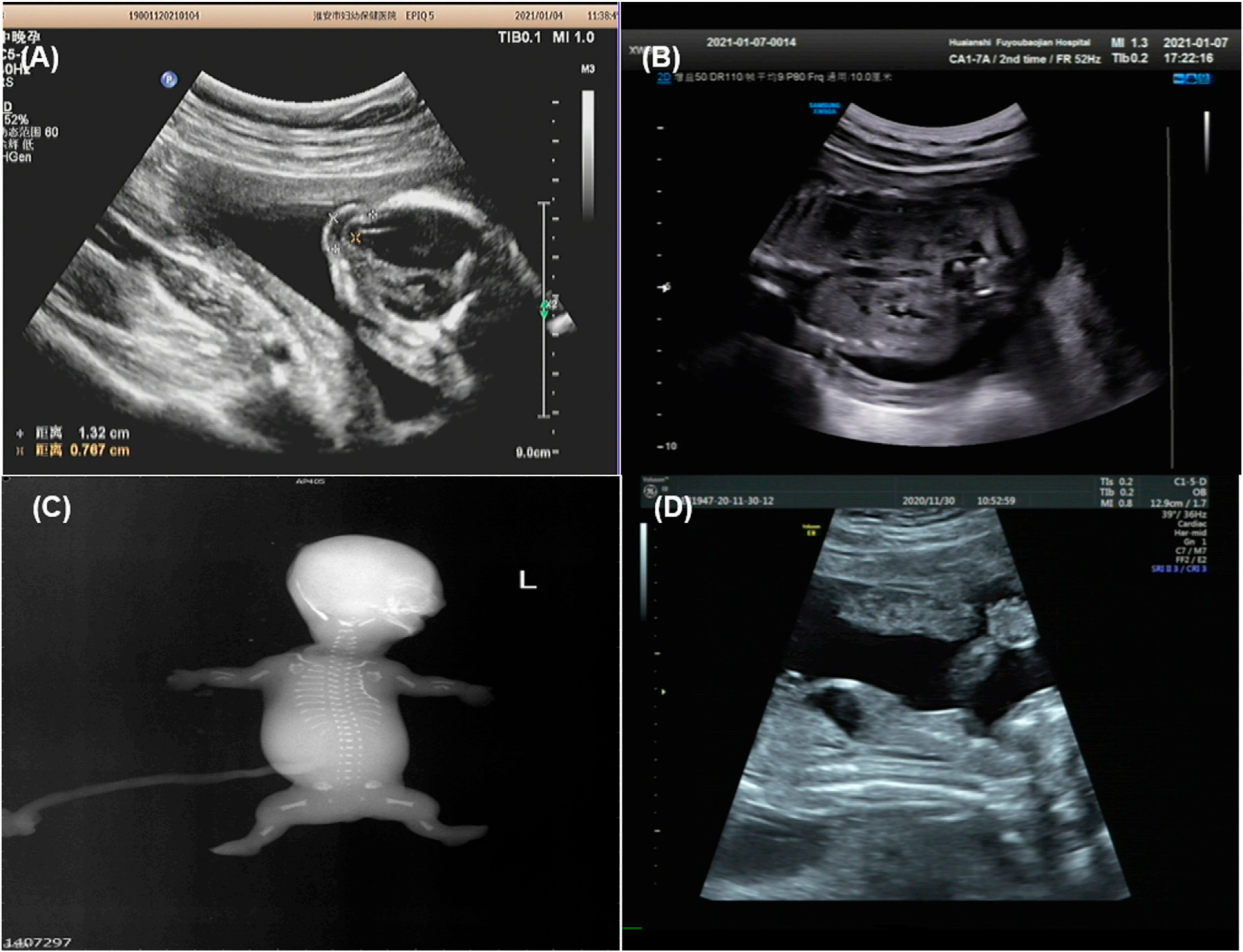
Imaging examination of ciliary diseases {Family 1 [(A): case 2], Family 3 [(B): case 5], Family 4 [(C): case 6], Family 5 [(D): case 8], occipital encephalocele (A), increased echogenicity in both renal cortexes (B), fetal X-ray showing bilateral shortened curved femora and disproportionately shortened tibiae (C), bone dysplasia and narrow chest (D)}.
